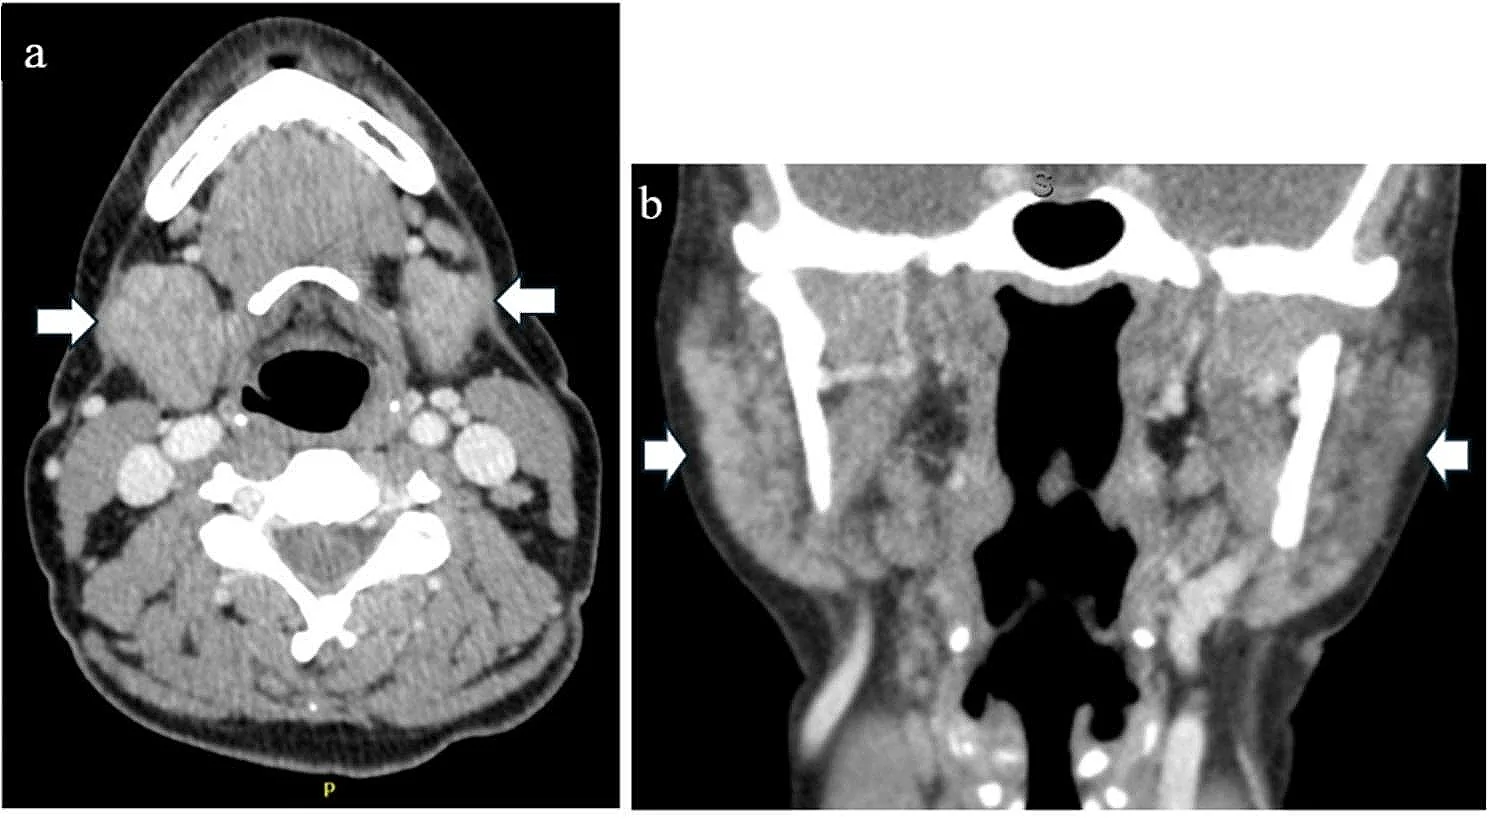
Representative radiological images of salivary gland lesions. (a) Submandibular gland enlargement (*white arrows*) of axial computed tomography (CT). (b) Parotid gland enlargement (*white arrows*) on coronal CT. Courtesy of Dr. Rafael Alves Cordeiro and Dr. Henrique Ayres Mayrink Giardini
